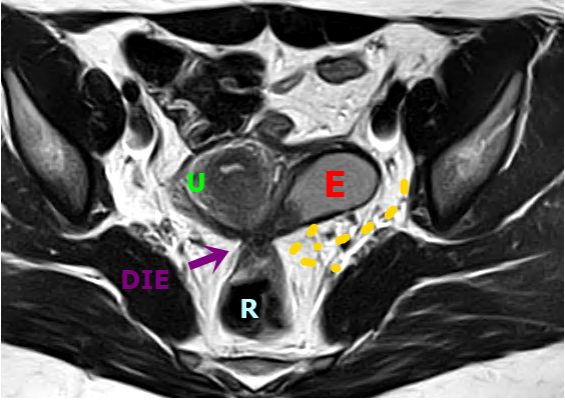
Axial T2W MR image shows a deep endometriosis (DE) plaque in the posterior uterus with adhesions that extend from the torus uterus, invading the wall of the rectum and promoting retraction and medialisation of the left ovary that contains endometrioma (E). Bowel-invasive endometriosis of the rectum is also present with a “mushroom cap” lesion. U: Uterus, E: Endometrioma, R: Rectum, DIE: DE plaque.
